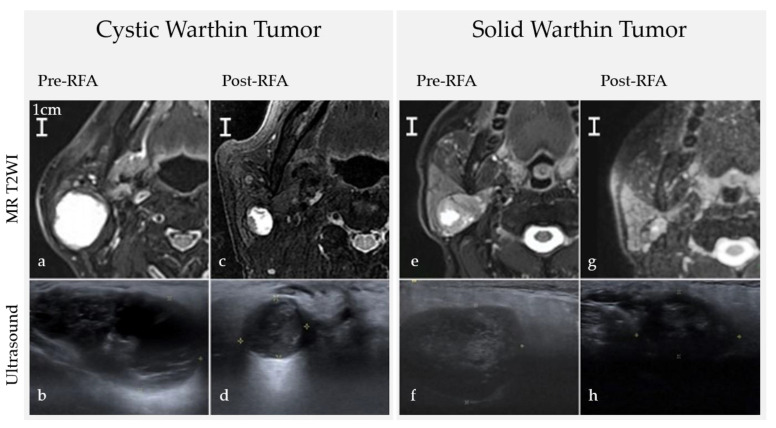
Images before and after RFA comparing consistencies and locations. (a,b) A huge right cystic Warthin tumor (35.47 mL) (c) Post-RFA 8 months VRR = 93.3%. (d) Post-RFA 1 months VRR = 68.8%. (e,f) A right solid Warthin tumor (18.13 mL) (g) Post-RFA 12 months VRR = 79.2%. (h) Post-RFA 1-month VRR = 51.5%. These two tumors were initially located in both superficial and deep lobes. After RFA, residual tumors were located in superficial lobes only. MR T2WI = Magnetic resonance T2-weighted image.
